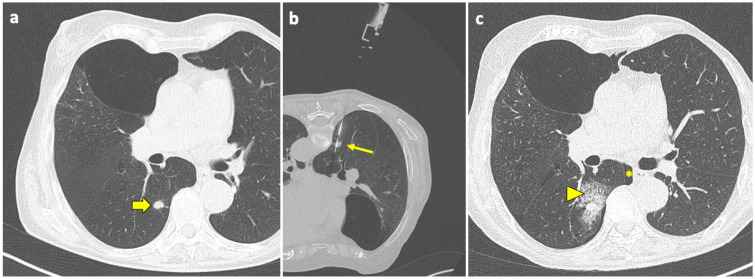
Post-procedural perilesional hemorrhage. (a) New solid pulmonary nodules are discovered during follow-up of a 73-year-old woman with a history of breast and rectal cancer, including one in the right lower lobe (thick arrow). (b) With the patient in a prone position, the sample notch of a CNB system (arrow) is positioned under CT guidance at the level of the nodule, and biopsy is performed. (c) At the end of the procedure, the patient is turned into the supine position and a final CT scan is performed, which shows a minor-degree pneumothorax (asterisk), along with a ground-glass opacity and septal thickening in the tissue around the nodule, consistent with localized hemorrhage (arrowhead). Both complications had no evolution or sequelae. Abbreviations—CNB: core needle biopsy; CT: computed tomography.
